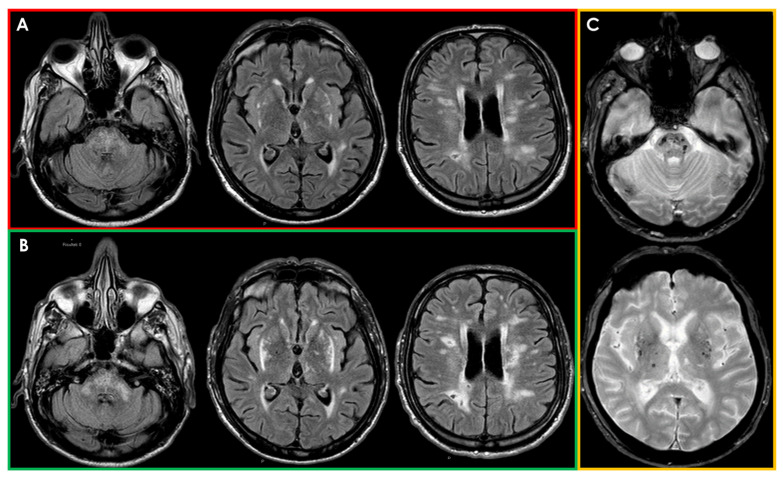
MRI of a patient with “classical” AFD. Panels (A,B) show axial FLAIR sequences highlighting the WMHs and lacunar burden at the baseline and after 1 year, respectively. In Panel (C), T2* sequence points out to the microbleeds’ burden in the pons and in the basal ganglia and external capsule.
